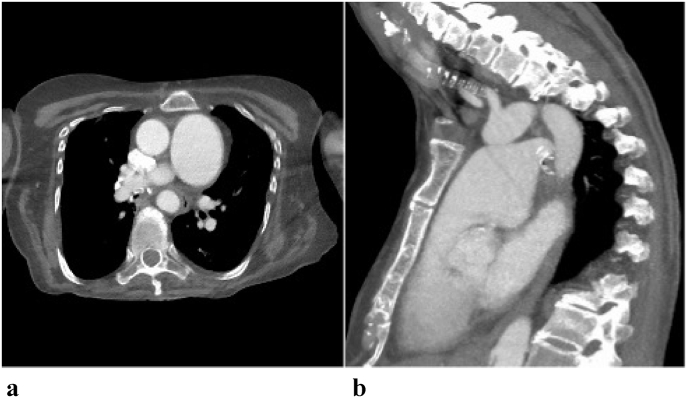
a (left): 1 mm axial view of CTA of the chest with and without contrast taken on initial presentation showing an aneurysmal dilatation of the main pulmonary artery measuring 5.0 × 6.4 cm. Patient was symptomatic with dyspnea on exertion at this time. Fig. 1b (right): Lateral view of initial CTA.
